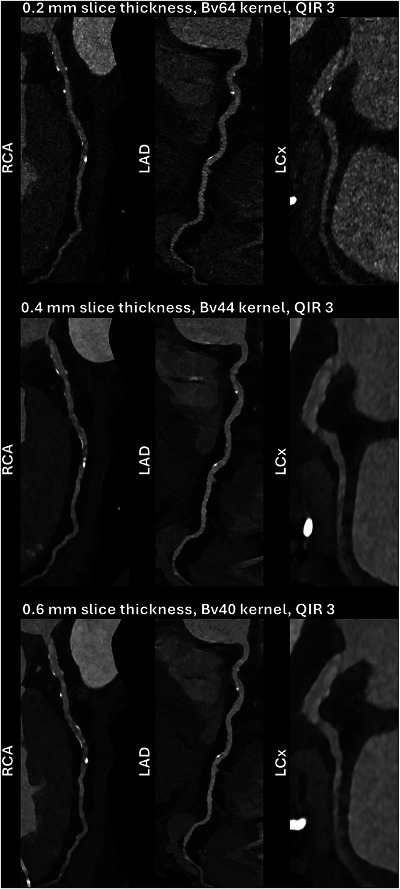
Comparison of the effects of slice thickness and convolution kernel on image impression using photon‐counting detector coronary computed tomography angiography in a 64‐year‐old male with a coronary artery calcium score of 252 Agatston units. LAD, left anterior descending artery; LCx, Left circumflex artery; QIR, quantum iterative reconstruction; RCA, right coronary artery.
